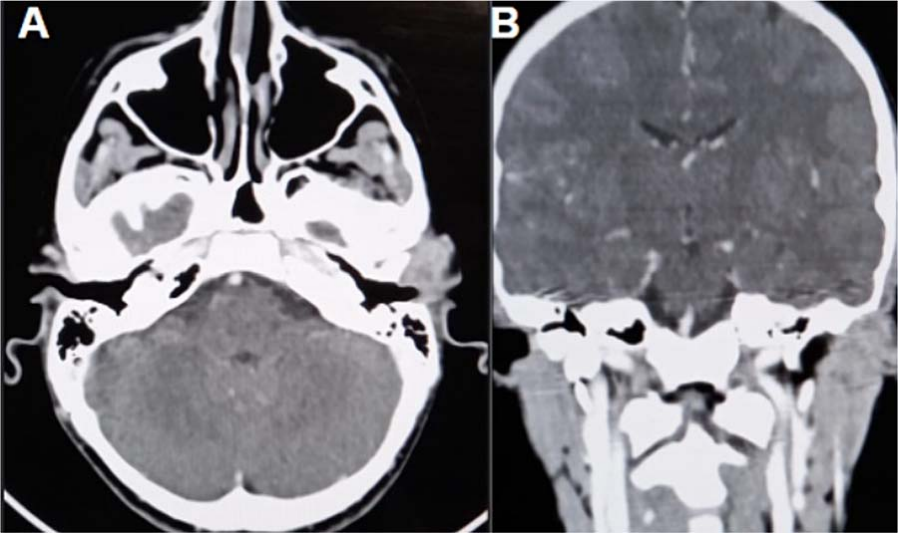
CT scan (A) axial section shows an ill-defined subcutaneous granulomatous process. (B) Coronal section of the process causing scalloping of the temporal bone.
